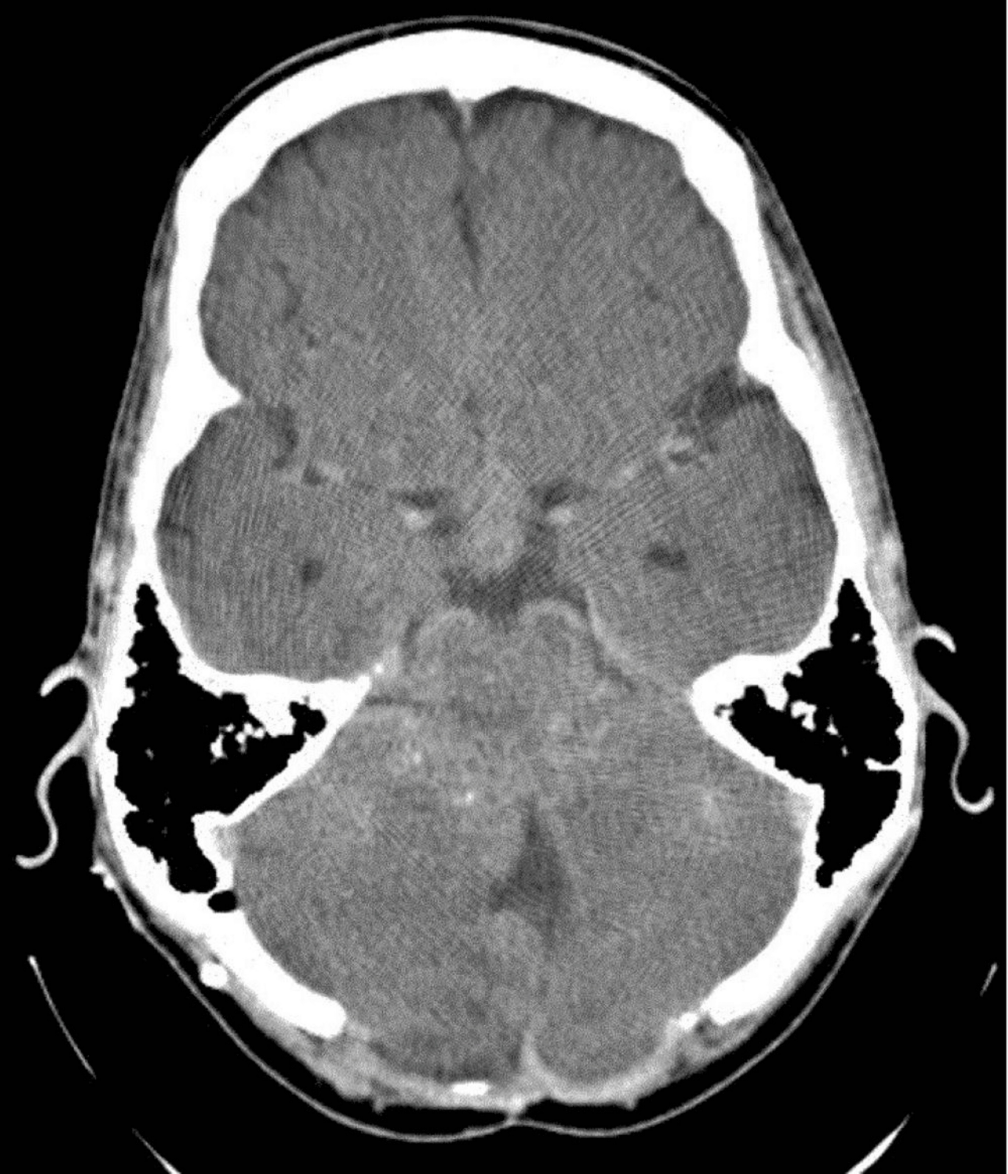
Follow-up brain CT performed six months after surgery.
